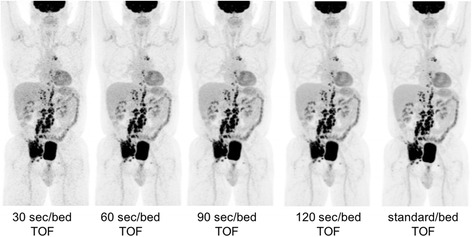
Images acquired 71 min after injection of 423.3 MBq of FDG and reconstructed at various times/bed (BMI 26.6). Reader 1’s scores for each time per bed reconstruction were, respectively, 4, 5, 5, 5, and 5; reader 2’s scores were 3, 4, 5, 5, and 5
